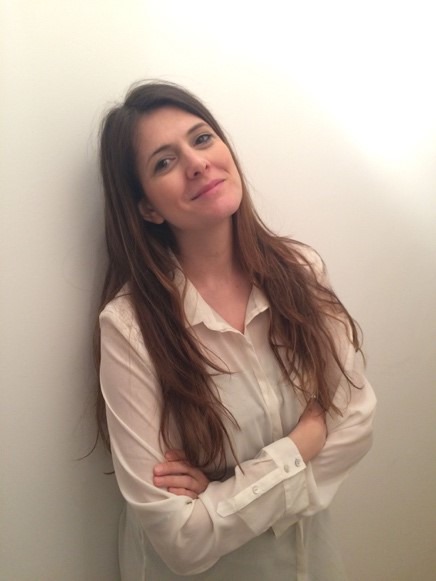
Dr. Vassiliki Pasoglou currently works as a radiologist at the Cliniques Universitaires Saint Luc in Brussels. She obtained her MD from the University of Ioannina, Greece, and following that she worked as a radiology resident at Université de Liège . After three years, she arrived at Université Catholique de Louvain in Brussels to commence her PhD thesis titled ‘’One step TNM staging of patients with high risk prostate cancer using MRI” [5]. Dr. Pasoglou was awarded the prestigious ESUI Vision Award 2015 for her work ‘’Whole-body 3D T1-weighted MR imaging in patients with prostate cancer: feasibility and evaluation in screening for metastatic disease’’. Her PhD thesis was completed in 2016, for which a summary is published in the current issue of the JBSR. She is currently engaged in research within the fields of oncological, abdominal, and whole body imaging at the Cliniques Universitaires Saint Luc.
